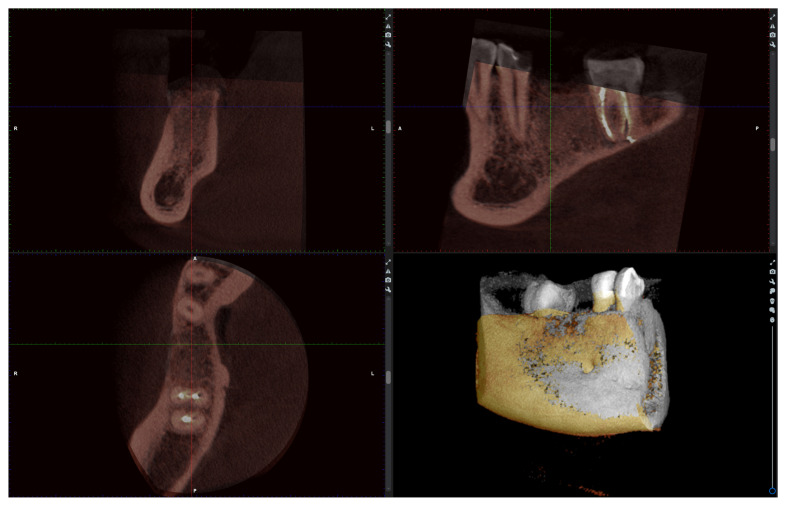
Radiographic superimposition.
